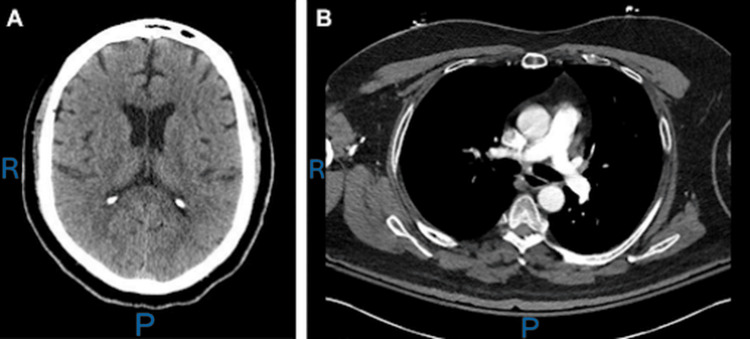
Radiologic imaging findings. (A) Axial computed tomography (CT) of the head demonstrates symmetric lateral ventricles with preserved gray-white differentiation and no evidence of acute intracranial hemorrhage, mass effect, or midline shift. (B) Axial CT pulmonary angiogram demonstrates normal contrast opacification of the main pulmonary artery and its right and left branches without evidence of pulmonary embolism. "R" denotes right-sided laterality and "P" denotes posterior direction.
